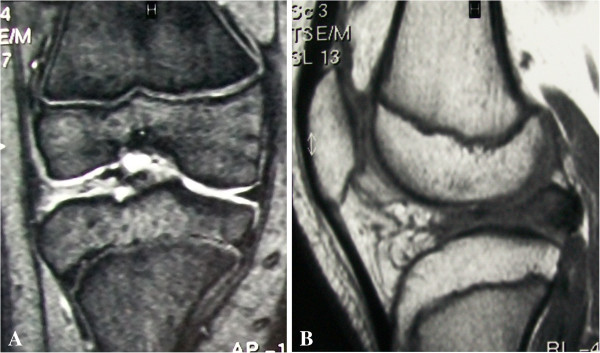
Coronal (A) and sagittal (B) MRI views of the right knee at 29 months follow-up. Note the discoid shape of the lateral meniscus in both coronal and sagittal views. The re-grown meniscus showed a different signal at MRI compared with the native one. Growth plates were still visible.
